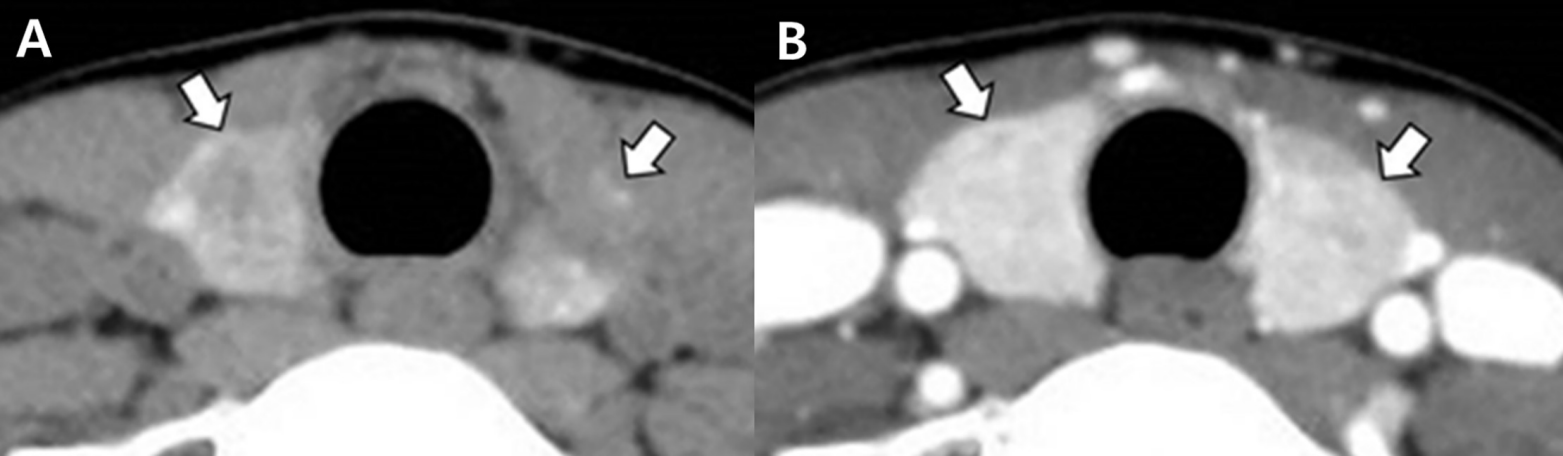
Computed tomography (CT) images in a 24-year-old woman with Hashimoto thyroiditis that was designated as “diffuse thyroid disease” CT category (papillary thyroid carcinoma in the right lobe). The thyroid gland (arrows) shows decreased and inhomogeneous attenuation in the non-enhanced CT image (A), and decreased and inhomogeneous enhancement in the contrast-enhanced CT image (B), but an anteroposterior diameter of 1–2 cm and a smooth margin in both the non-enhanced and contrast-enhanced CT images.
